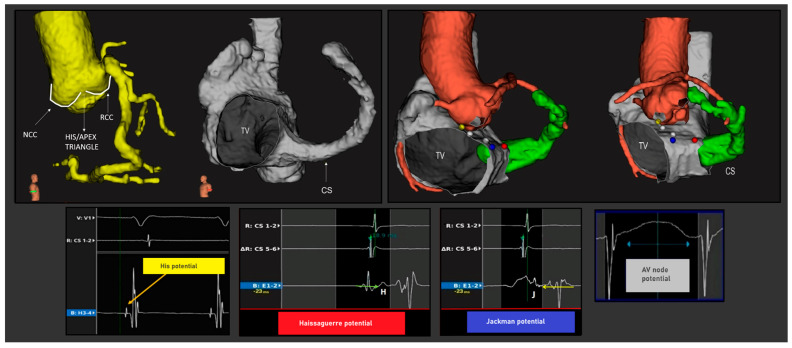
Merged acquisition from the 3D mapping and reconstruction of computed tomographic datasets, indicating different stages. Geometry import is available to import geometries created by another application (such as computed tomography or magnetic resonance imaging) and align it to the already created maps, such that maps can be compared to the imported geometry. The imported geometrical shells can be used as a reference, for example, to identify anatomical features in advance of mapping. In this example, a computed tomographic dataset for the segmentation of the aortic root and the coronary arteries was used, along with the right atrium including the coronary sinus. Once the electroanatomical map had been acquired, it was aligned with the reconstructed segmentations. This alignment allows us to identify the aortic root relative to Koch’s pyramid and correlated AV nodal structures and electrograms, listed as follows: His (yellow tag), AV node (white tag), Jackman potentials (red tag) and Haissaguerre potentials (blue tag) are shown. NCC = non coronary cuspid, RCC = right coronary cuspid, TV = tricuspid valve, CS = coronary sinus.
